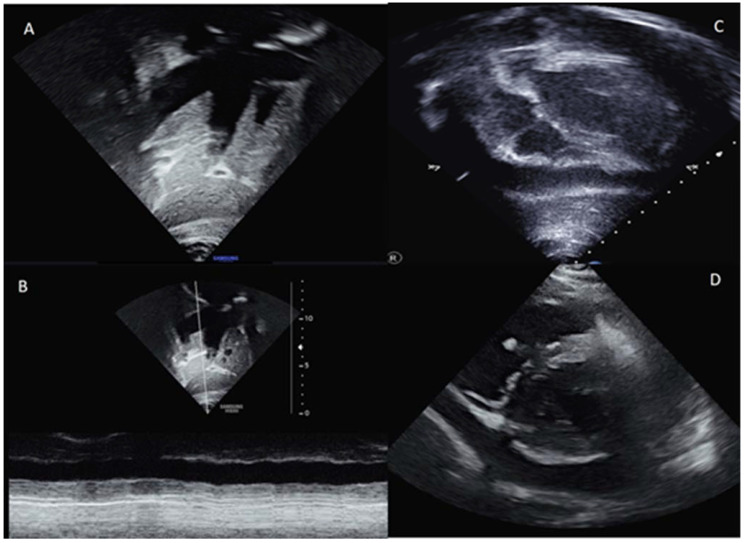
Echocardiogram images showing inferior vena cava and suprahepatic veins dilation (A); severe reduction of the normal inferior vena cava collapse (B); severe pericardial effusion in subxiphoid (C) and short axis view (D).
